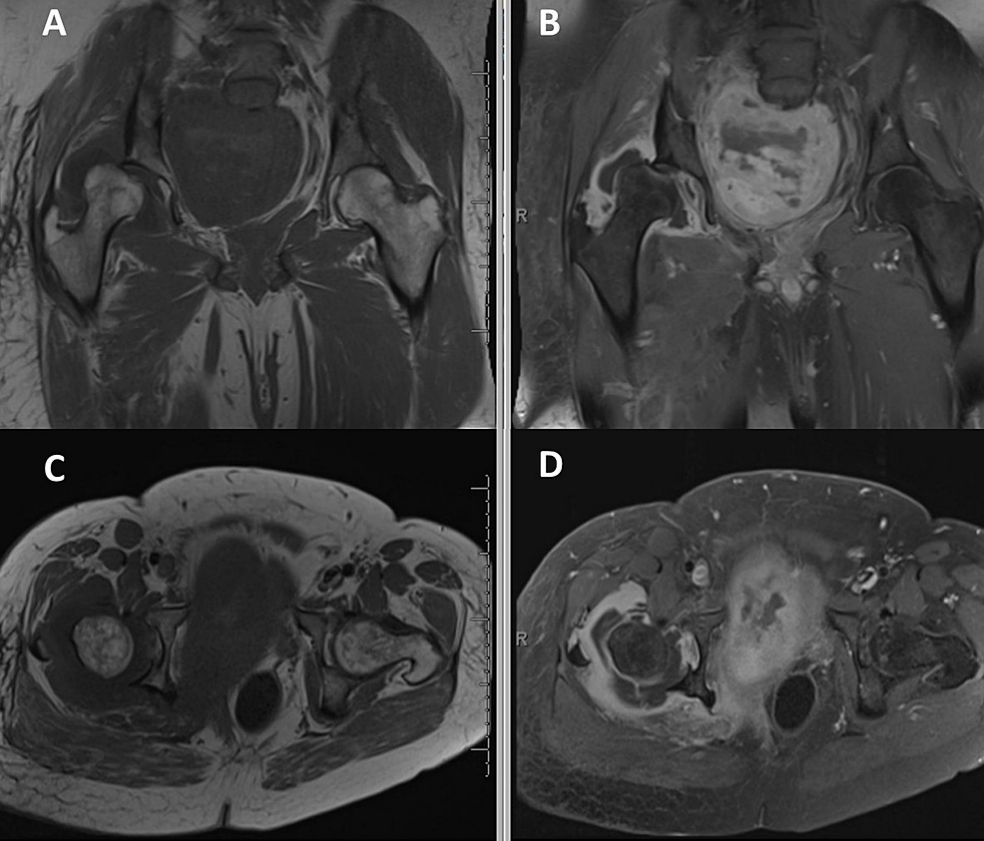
Pre and post-contrast T1-weighted pelvic MRI with coronal (A and B) and axial (C and D) images at the level of the hip. These images demonstrate an intra-articular/periarticular lesion that leads to a shallow acetabulum, resulting in the femoral head's dislocation.
